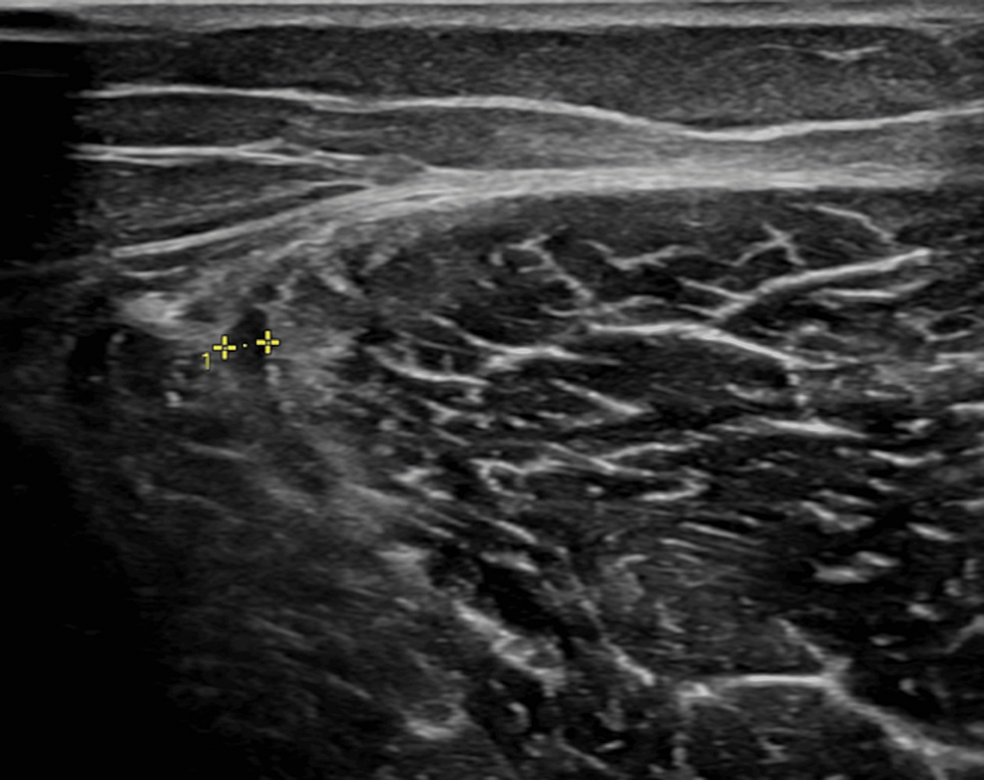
Musculoskeletal ultrasound of the left thigh: recurrent injury, with a rupture area of the peripheral aponeurosis of the distal junction of the long head of the biceps femoris (BF) (yellow *), associated with subaponeurotic edema and perifascial fluid lamina.
